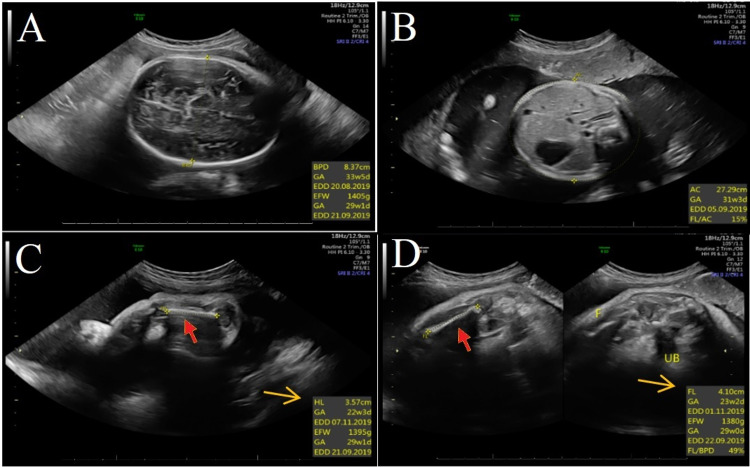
Fetal ultrasound: A) HC; B) AC; C) HL; D) FL. AC: abdominal circumference; HL: humerus length; BPD: biparietal diameter; EDD: estimated date of delivery; EFW: estimated fetal weight; F: fetus; FL: femur length; FL/AC: femur length to abdominal circumference ratio; GA: gestational age; HC: head circumference; UB: urinary bladder.
